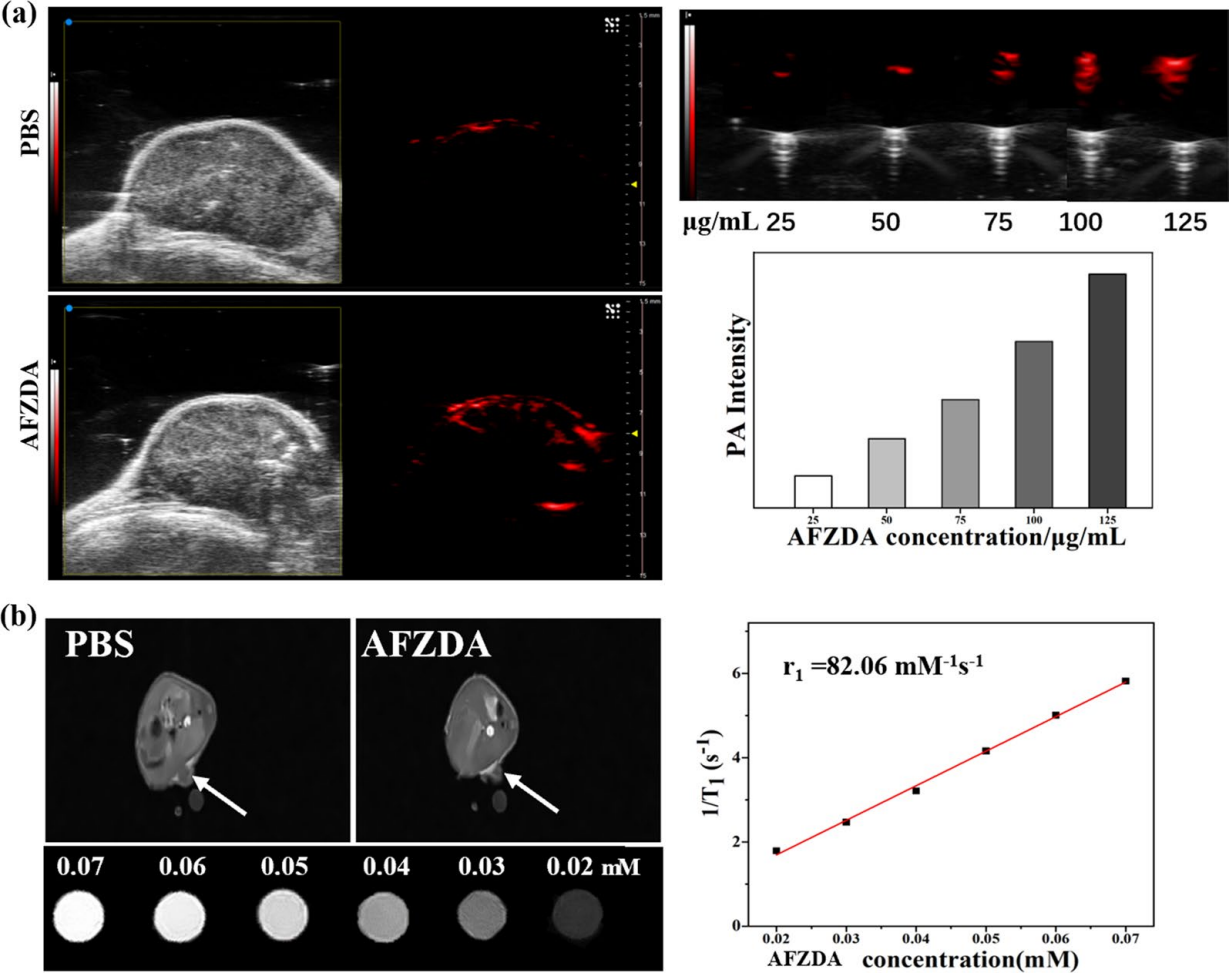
a The PA images of PBS and AFZDA NPs, and the quantitative PA signals of AFZDA NPs with concentrations were analyzed.  b The MR images of PBS and AFZDA NPs in vivo and T1-weighted magnetic resonance imaging of AFZDA NPs with concentrations in vitro, and T1 relaxation rate (r_1_) of AFZDA NPs were measured
